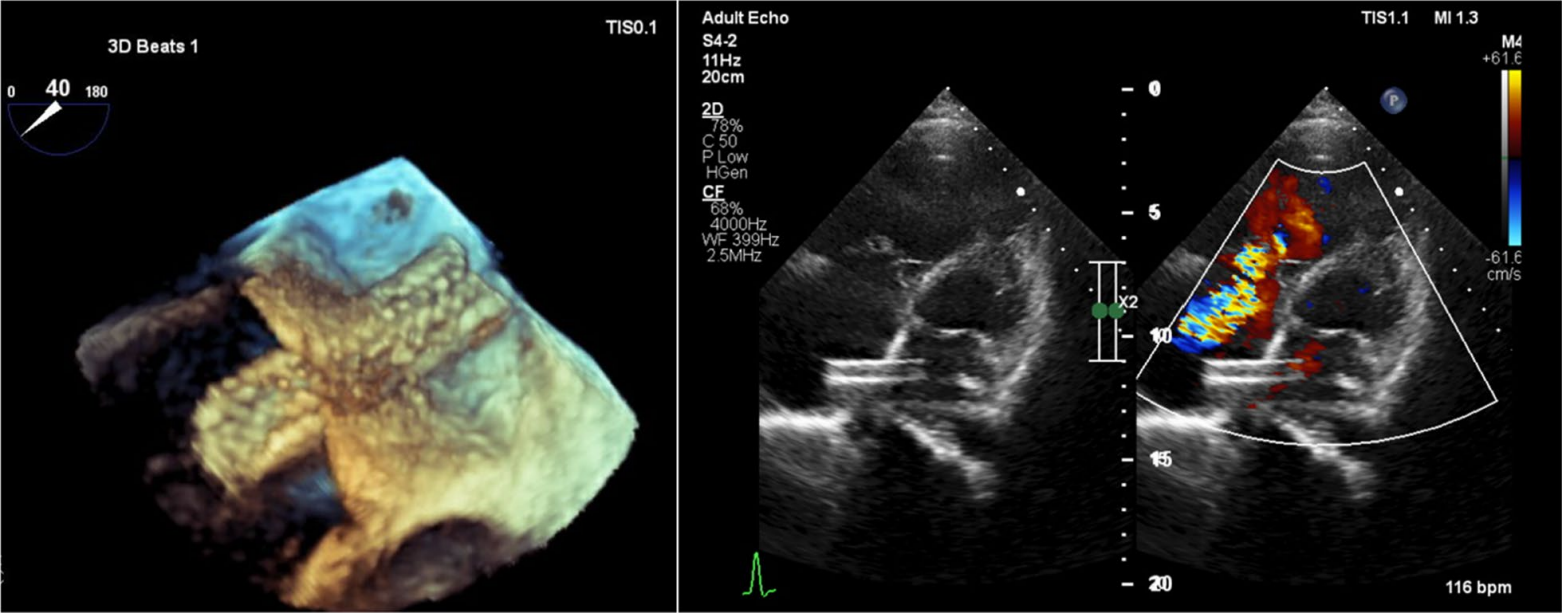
The position of the IAS stent as seen by echocardiography
